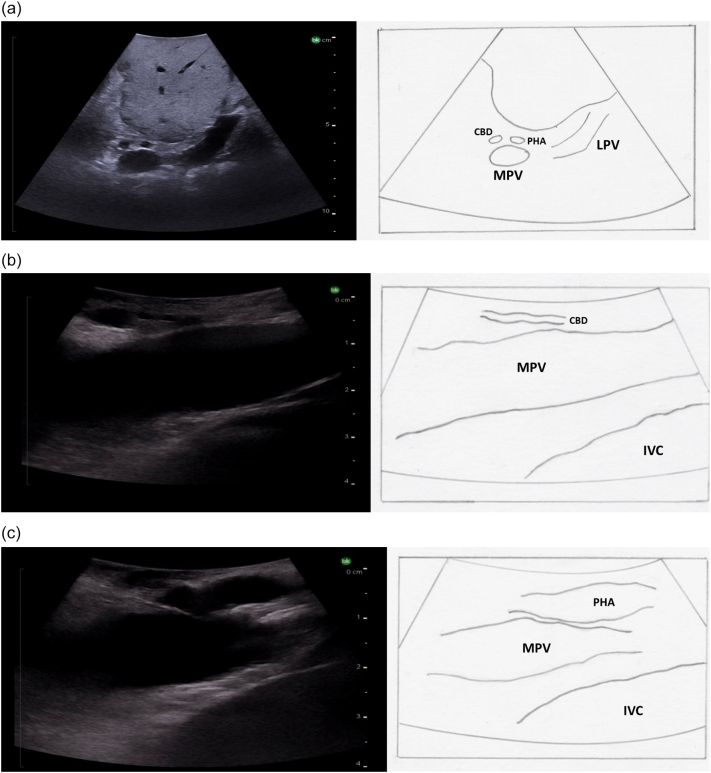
Intraoperative ultrasound of the hepatoduodenal ligament. Ultrasound image with the probe in the transverse orientation (a). Placing the probe longitudinally over the lateral aspect of the hepatoduodenal ligament visualizes the bile duct (b) and sliding the probe medially will visualize the proper hepatic artery. MPV main portal vein, PHA proper hepatic artery, CBD common bile duct, LPV left portal vein, IVC inferior vena cava.
